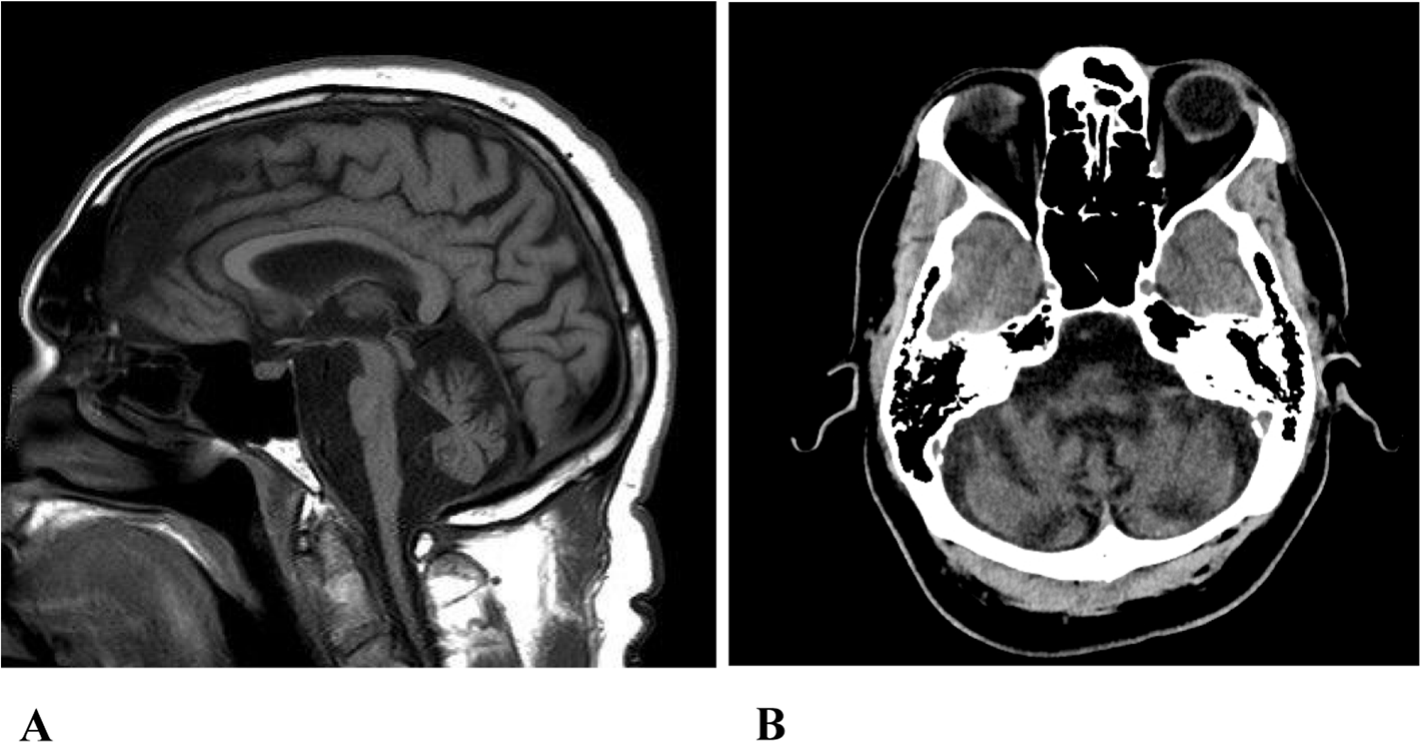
a Sagittal T1-weighted MRI brain demonstrating atrophy of the brainstem and cerebellum with increment in cerebellar folia. b. Axial CT head without contrast  demonstrating atrophy of the cerebellar hemispheres with prominent sulci.
